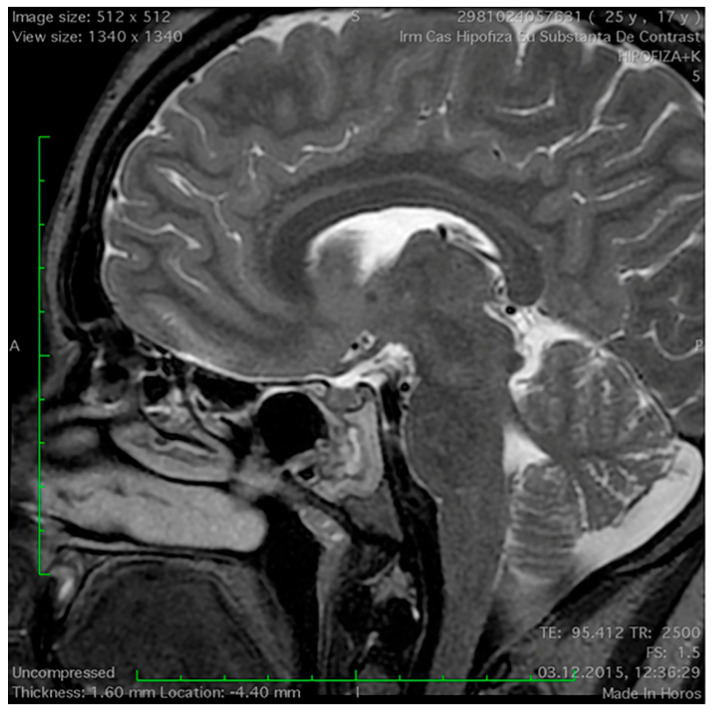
Mucosal thickening of the sphenoid sinus on a sagittal T2−weighted magnetic resonance imaging section in a patient with pituitary adenoma.
